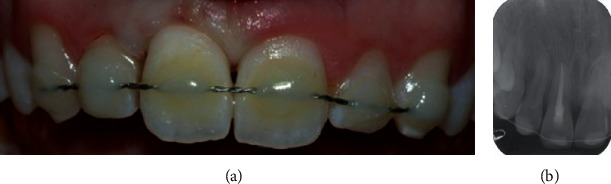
(a) Clinical and (b) radiographic view of the upper incisor after reimplantation and splint.
